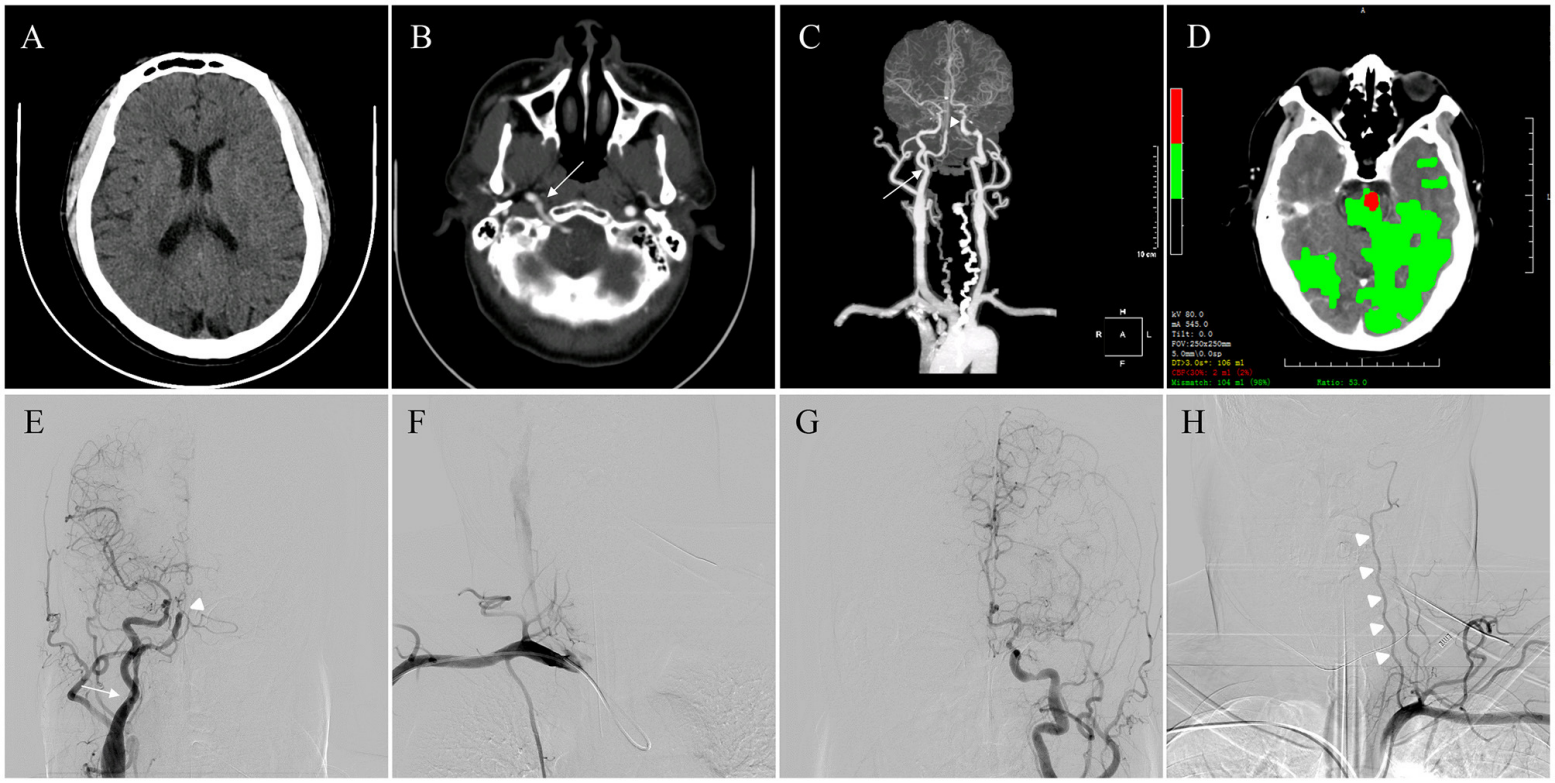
Preoperative multimodal neuroimaging evaluation. (A) Intracranial hemorrhage was excluded through computed tomography (CT). (B) Cross-sectional contrast CT revealed abnormal anastomosis (arrow) between the internal carotid artery (ICA) and the intracranial segment of the vertical artery (VA) located in the atlantooccipital space. (C) CT angiography (anterior-posterior view) showed basilar artery occlusion (arrowhead) and that right VA (arrow) originated from the ICA. (D) Volumes of infarct core (red area) and penumbra (green area) were evaluated automatically from CT perfusion to calculate the mismatch volume. (E) Right hemispheric angiography showed complete occlusion of the middle segment of the basilar artery (arrowhead) and that persistent hypoglossal artery (PHA) (arrow) originated from the right ICA. (F) Right subclavian artery angiography showed the absence of VA origin. (G) Left middle cerebral artery occlusion was observed with collateral vessel formation. (H) Slender left VA (arrowheads) demonstrated in left hemispheric angiography.
